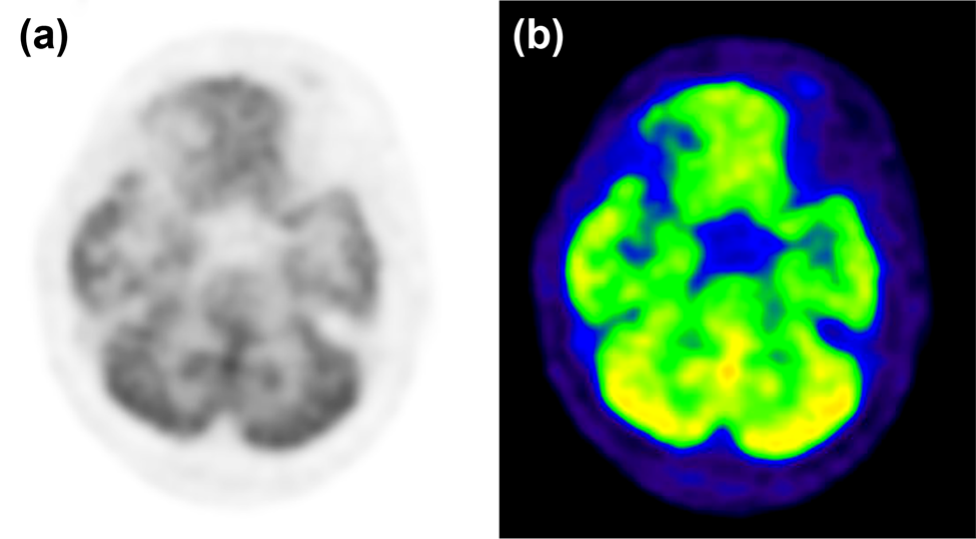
PET-CT (a) and ^18^F-FDG (b) did not reveal any obvious abnormalities.
